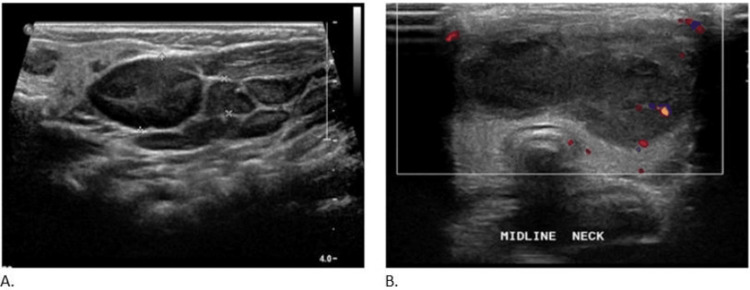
(A) Left lateral ultrasound and (B) midline neck ultrasound The images show an anterior midline neck collection which is multi-septated with thick walls and internal debris. It measures 5.5 x 1.5 x 4 cm with a total volume of 17.5 ml.
